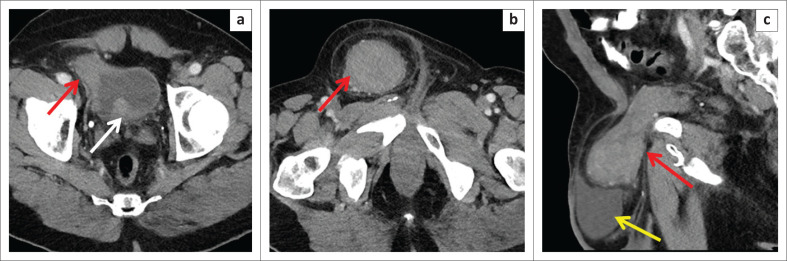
Computed tomography (CT) scan of the pelvis with intravenous contrast: (a) Axial CT image of the pelvis acquired during the portal venous phase demonstrates irregular thickening of the right anterolateral urinary bladder wall (red arrow) and an enlarged prostate bulging into the bladder base (white arrow). (b) Axial and (c) sagittal CT images of the inguinal region reveal a heterogeneous, enhancing soft tissue mass in the right inguinal canal, inseparable from the right anterolateral aspect of the urinary bladder wall (red arrow). There is a moderately sized hydrocoele in the right scrotum (yellow arrow).
